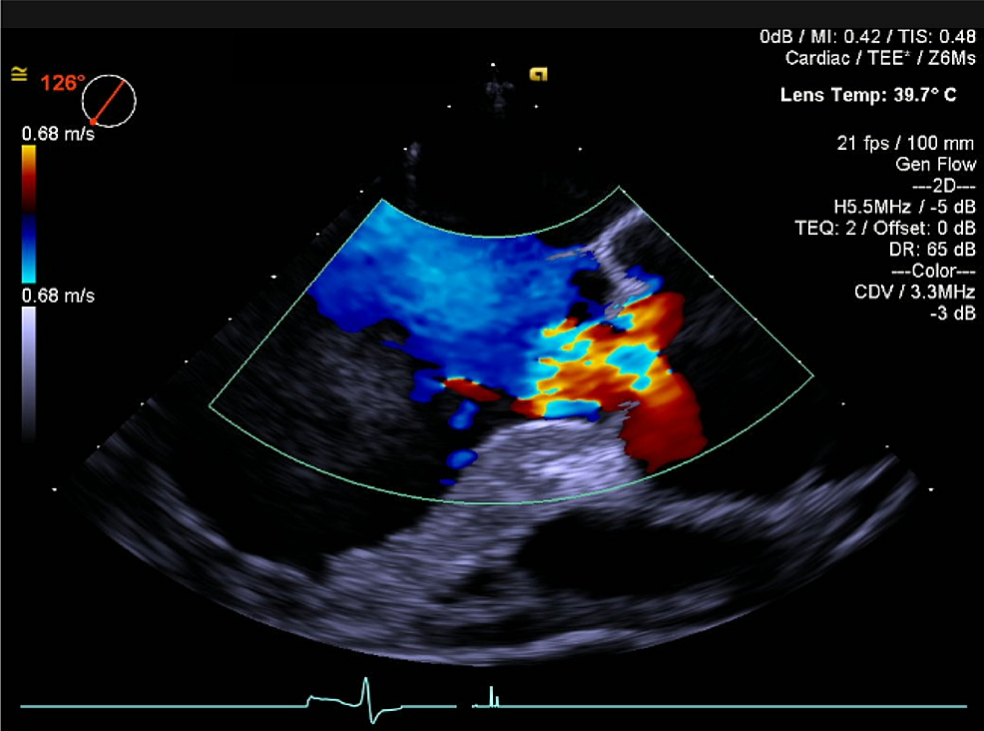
Transesophageal echocardiography during surgery
